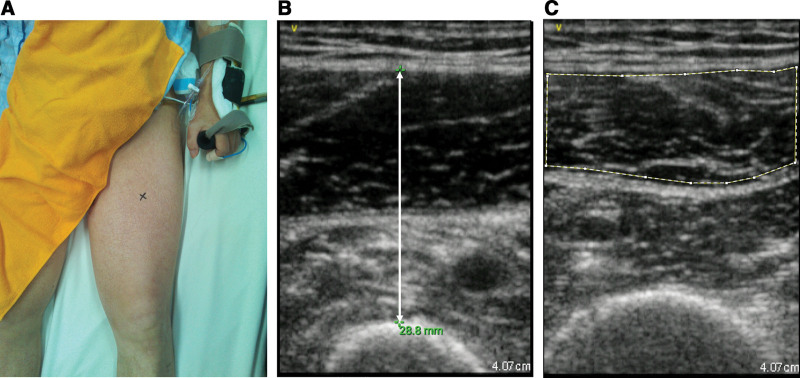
Measurement by ultrasound imagery. (A) Measurement site; (B) measurement of muscle thickness; (C) measurement area of echo intensity.
